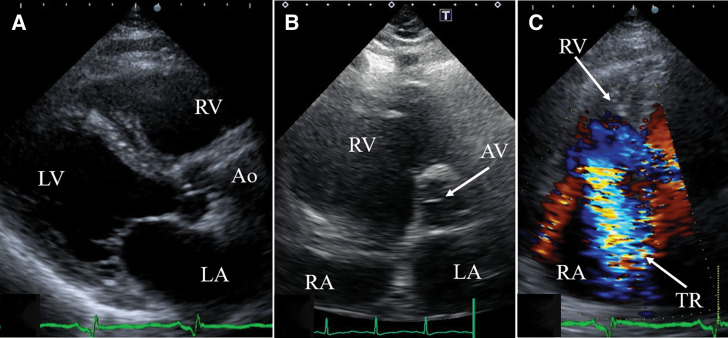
Transthoracic echocardiographic findings. Parasternal long-axis view (A) and short-axis view (B) demonstrate right ventricular enlargement. The apical view (C) demonstrates severe tricuspid regurgitation. Ao, aorta; AV, aortic valve; LA, left atrium; LV, left ventricle; RA; right atrium; RV, right ventricle; TR, tricuspid regurgitation
